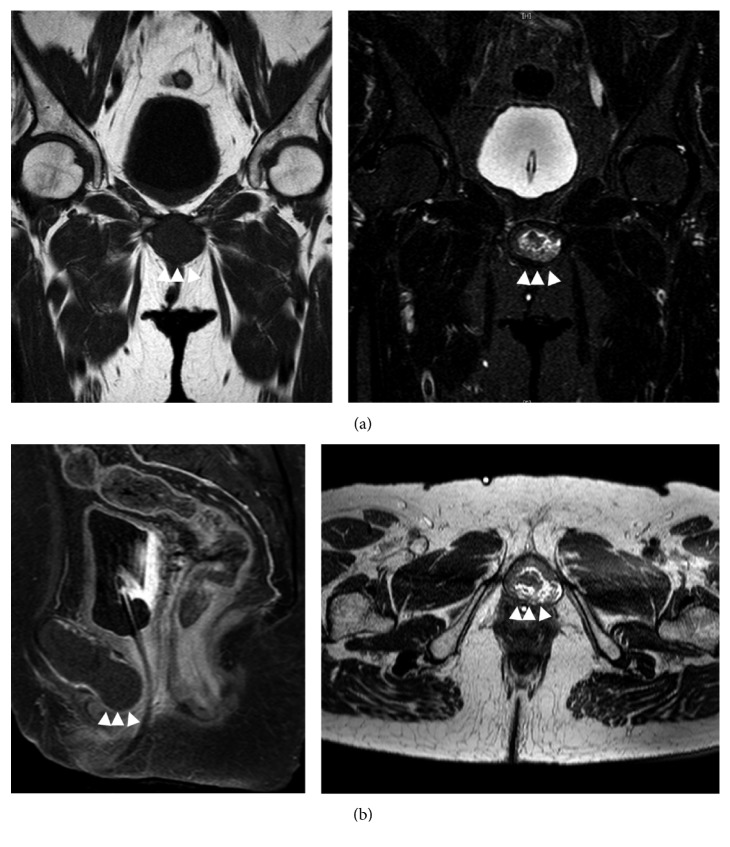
Magnetic resonance imaging (MRI) findings. (a) Coronal plane, noncontrast, T1-weighted, STIR images (△△△, subpubic cyst). (b) Sagittal plane, gadolinium-enhanced, T1-weighted image, showing compression of the ureter from the anterior, and axial plane T2-weighted image.
